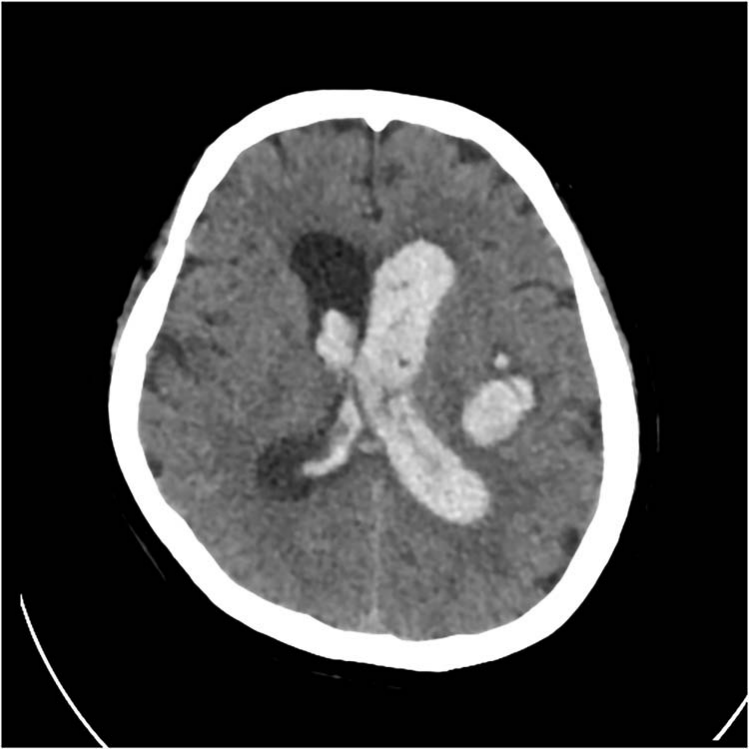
Initial CT revealing acute left-sided intraparenchymal haemorrhage with intraventricular extension and mass effect.
